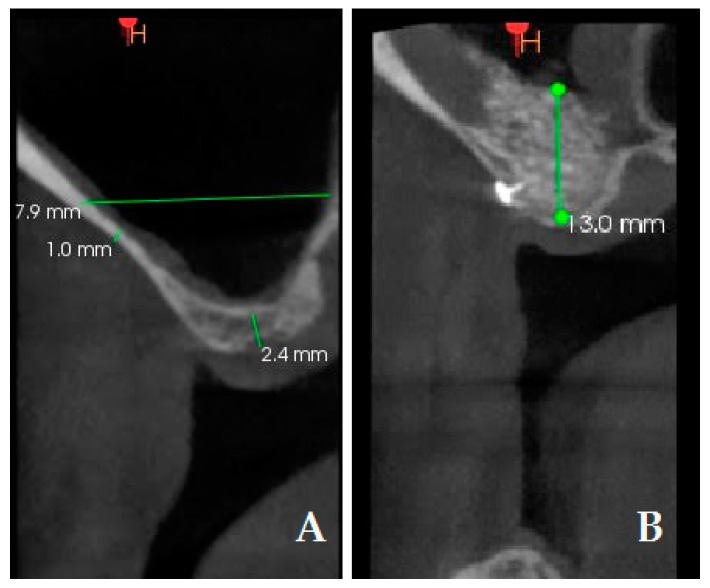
Cone Beam Computed Tomography (CBCT) measurements: preoperative residual bone (2.4 mm); preoperative thickness of lateral bone in the center (1 mm) (A); postoperative bone height (13 mm). A bone tack is observable in the postoperative CBCT (B).
